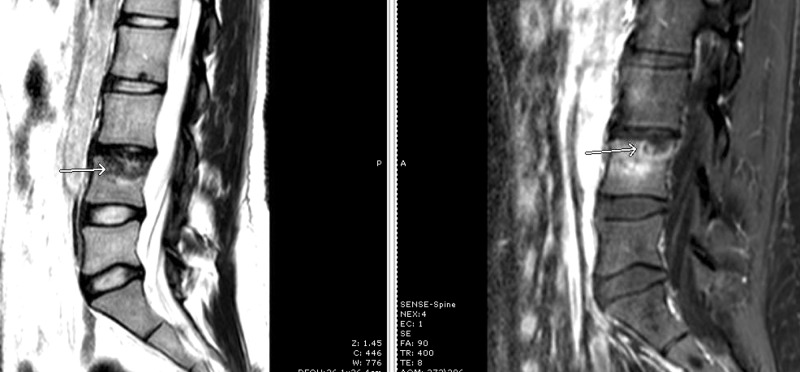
Initial MRI lumbar spine Magnetic resonance imaging (MRI) sagittal view with T2-weighted (left) and contrast-enhanced T1-weighted (right) sequences showing signal abnormality in the superior endplate of the L4 vertebral body, initially interpreted as a Schmorl's node (arrows).
